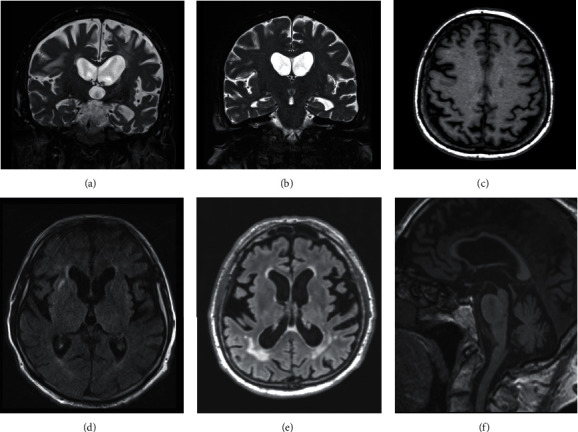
Overview of typical MRI findings in our cases. Asymmetrical atrophy of the opercular and medial temporal lobe was seen in nfvPPA patients ((a) cases 1, 2, 3, and 6; in case 4, temporal atrophy predominated on the right side) and in case 9 with lvPPA. Early left-side anterotemporal atrophy was detected in svPPA patients ((b) cases 7 and 8). In contrast, parietal atrophy of various degrees was rather symmetric in our cases (c), except in case 8. Leukoaraiosis ranged from very mild ((d) Fazekas grade 1) to severe ((e) Fazekas grade 3). GGT comorbidity was associated with atrophy of the mesencephalon (“hummingbird sign”) and with callosal atrophy ((f) cases 3, 6, and 7).
